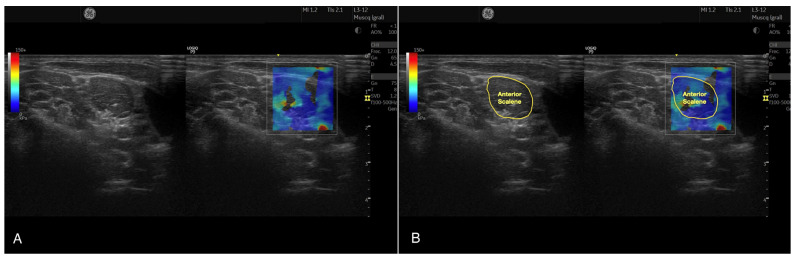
Raw B-mode Ultrasound and Shear Wave Elastography image acquired at C7 level for assessing the anterior scalene muscle (A) and muscle contouring for acquiring the stiffness metrics (B).
